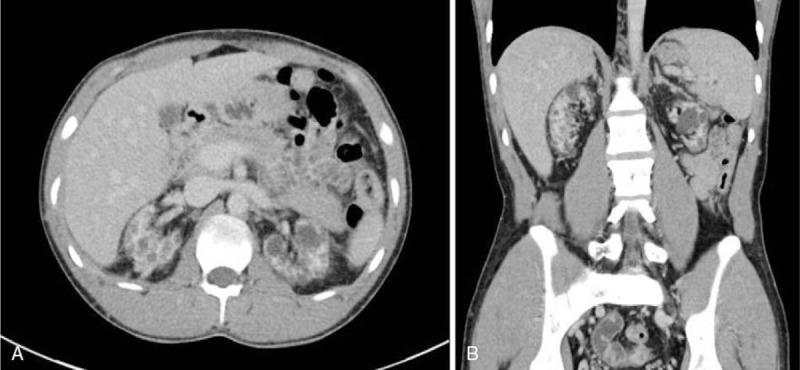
Three-month follow-up CT scan showing complete resolution of right perirenal hematoma. (A) Axial view. (B) Coronal view.
